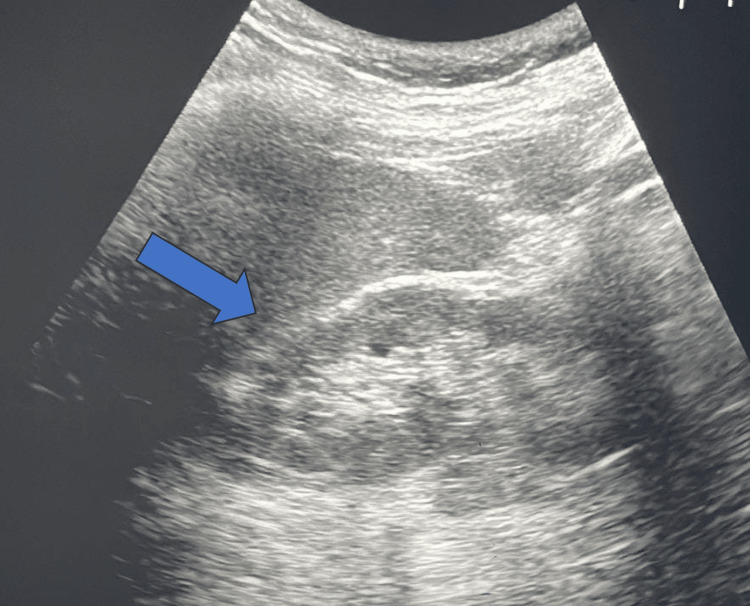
Ultrasonography of the abdomen was suggestive of normal shape, size, and echotexture of the kidneys (blue arrow)
